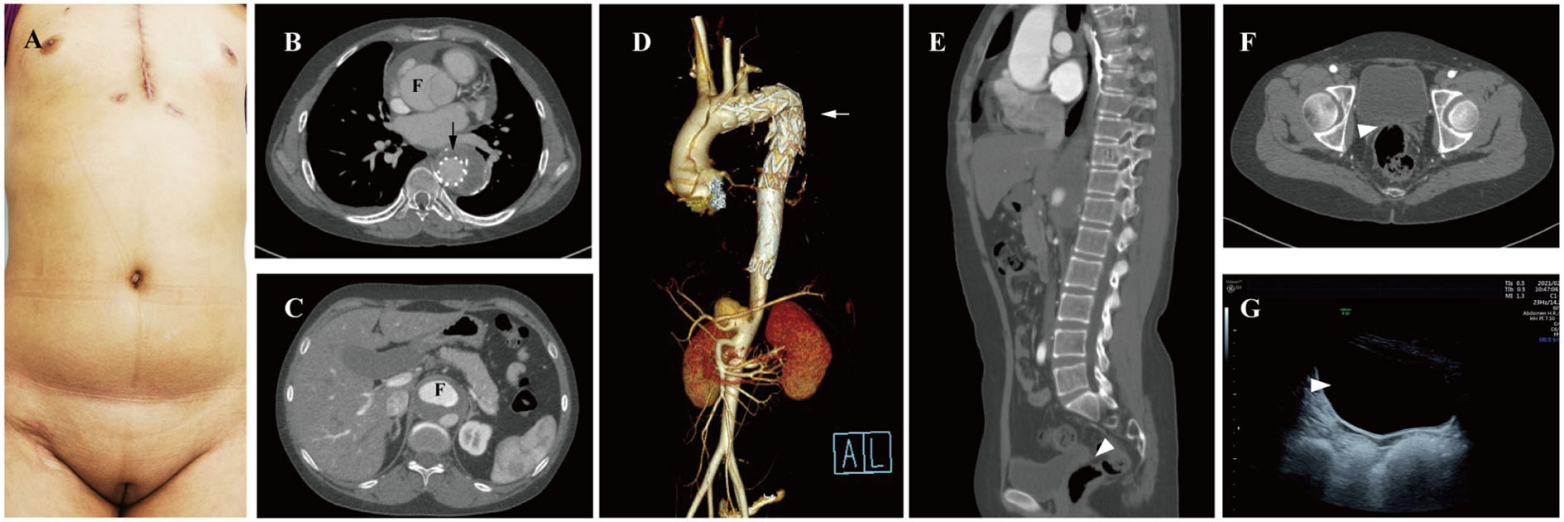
Preoperative examination of the 25-year-old female patients. (A) Undeveloped secondary sexual characteristics. CTA shown aortic dissection involving the ascending (B) and abdominal aorta (C), F, false lumen. (D) Thoracic endovascular aortic stent was grafted (arrow); no uterus or ovaries was found between urinary bladder and rectum by CTA (E,F, arrowhead) and transabdominal ultrasound (G, arrowhead). CTA, computed tomography angiography; F, false lumen.
